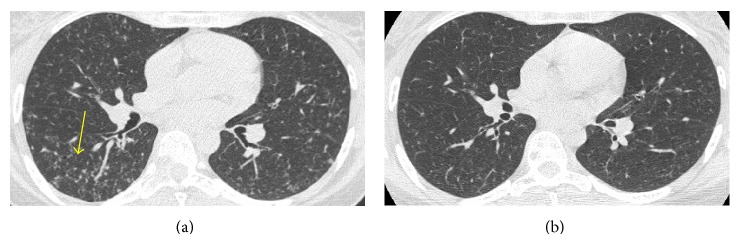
(a) CT of the chest with evidence of bilateral and symmetrical middle lung zone nodules of irregular appearance and cysts. (b) Repeat CT chest 18 months later with disappearance of the nodules and cysts bilaterally.
